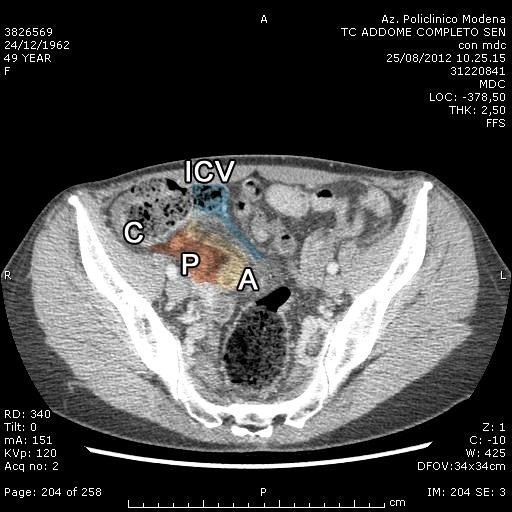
CT scan with contrast medium showing a bent phlegmonous appendix with a swollen tip enclosed by an inflammatory plastron tenaciously adherent to the last ileal loop and to the right fallopian tube fimbria [A = appendix; C = caecum; ICV = ileo-caecal valve; P = plastron].
